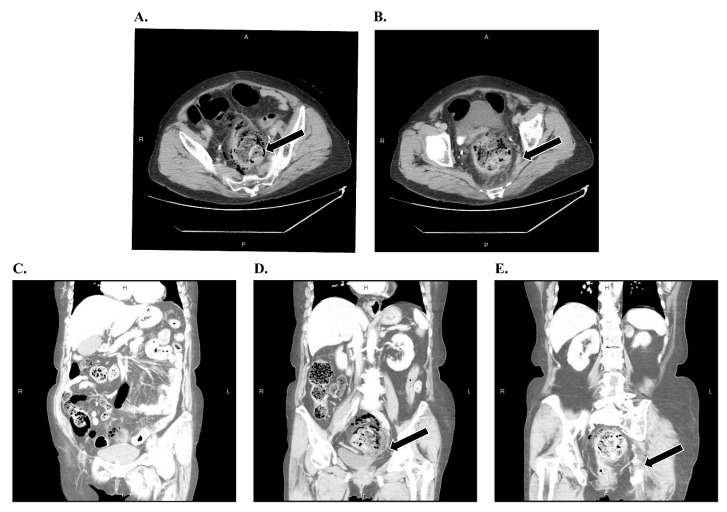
(A–E) Contrast-enhanced CT imaging demonstrating extraluminal gas (arrows) in the perirectal space, indicating full-thickness ischemic rectal perforation.
